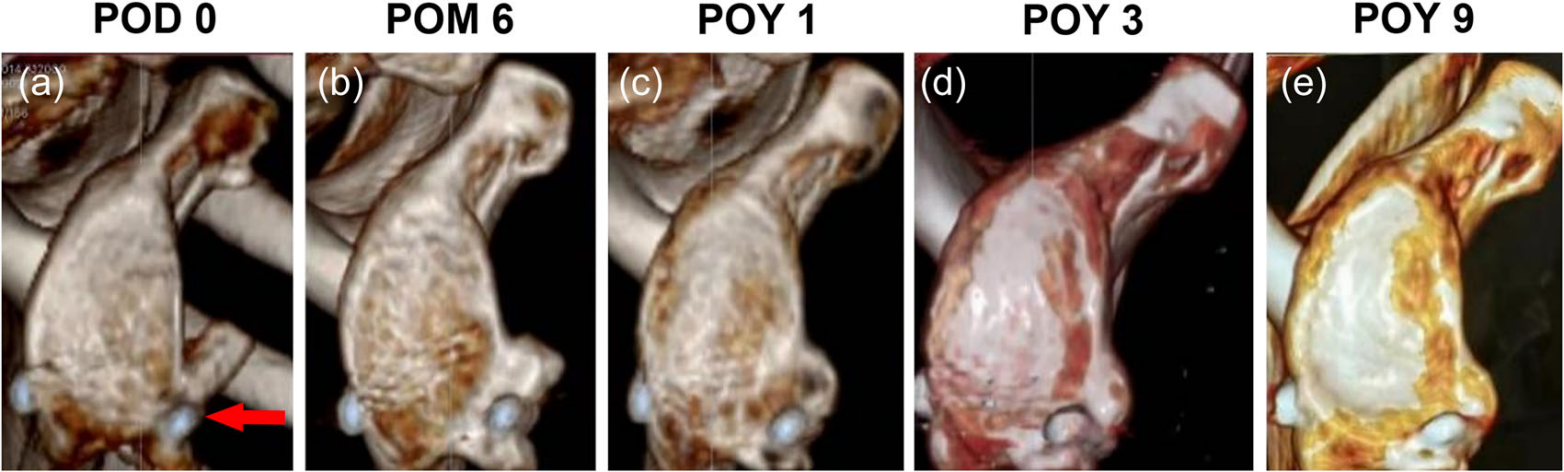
One case (No. 1) of CG fracture during the surgery (3D CT en‐face sagittal view of the glenoid). (a) The red arrow showed the CG fracture at POD 0. (b) The CG‐glenoid surface healed at POM 6. (c–e) The CG‐glenoid remodelling at different follow‐up time points. 3D, three‐dimensional; CG, coracoid graft; CT, computed tomography; POD, postoperative day; POM, postoperative month; POY, postoperative year.
